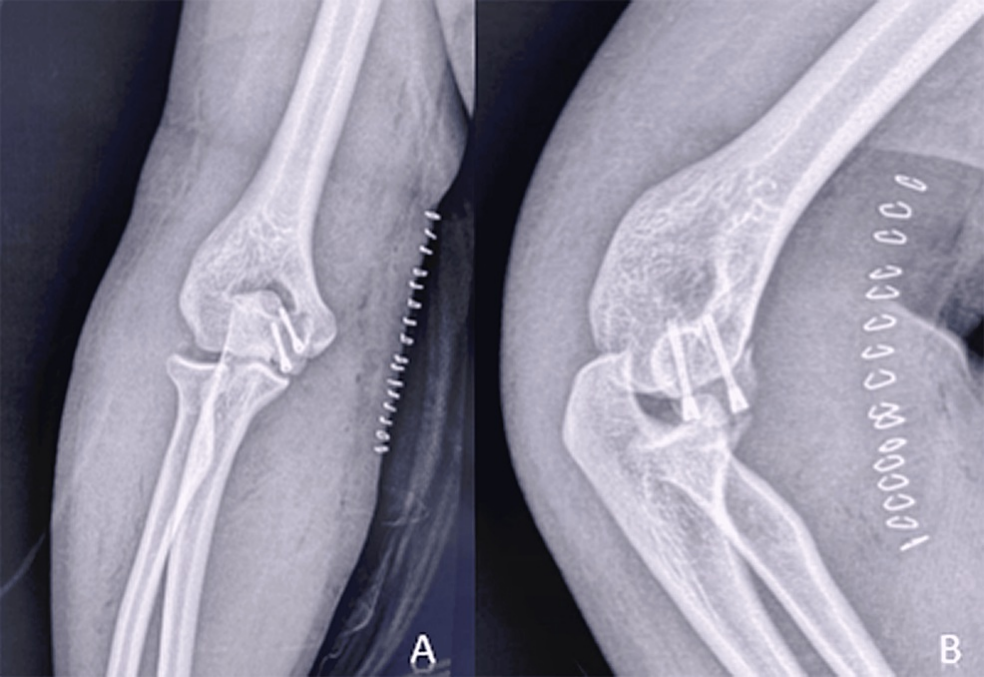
Postoperative frontal (A) and lateral (B) control X-rays showing the osteosynthesis in place
